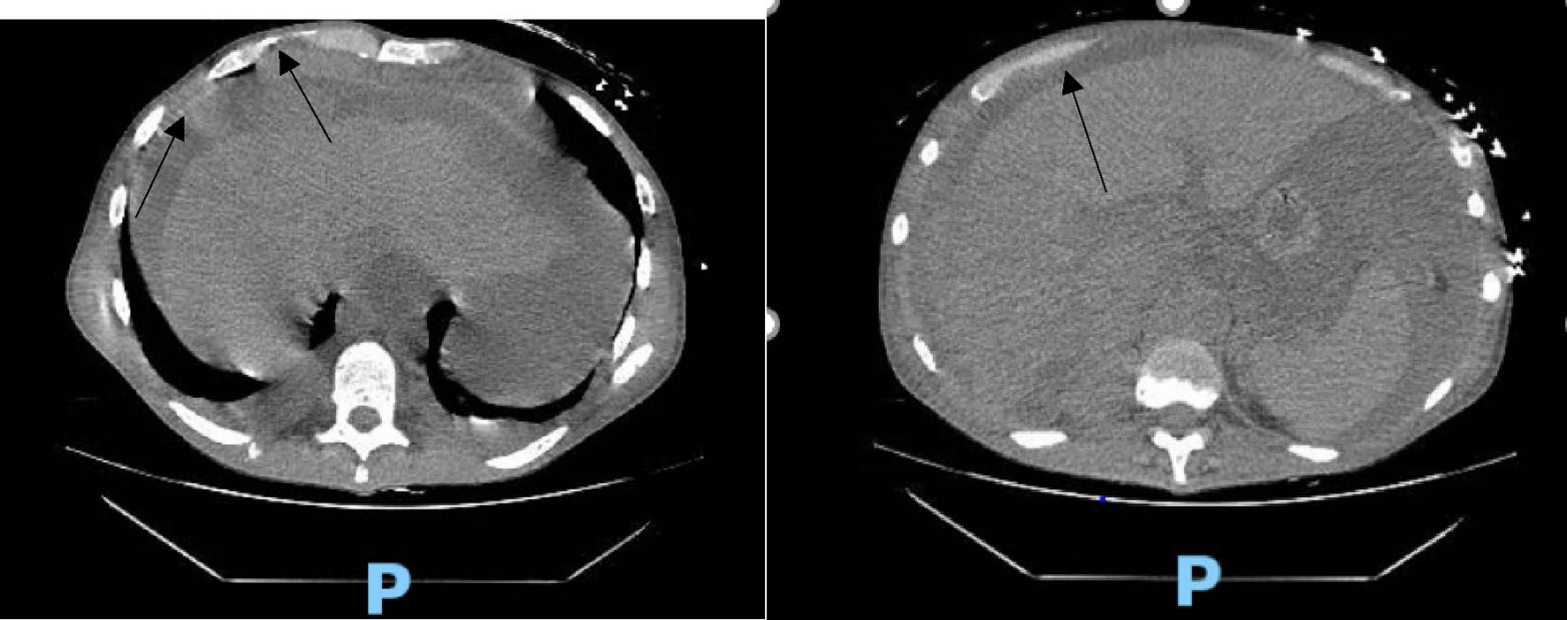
CT abdomen and chest showing heterogeneous liver, possible hepatic capsular implants, large volume ascites involving abdomen and pelvis and omental caking
